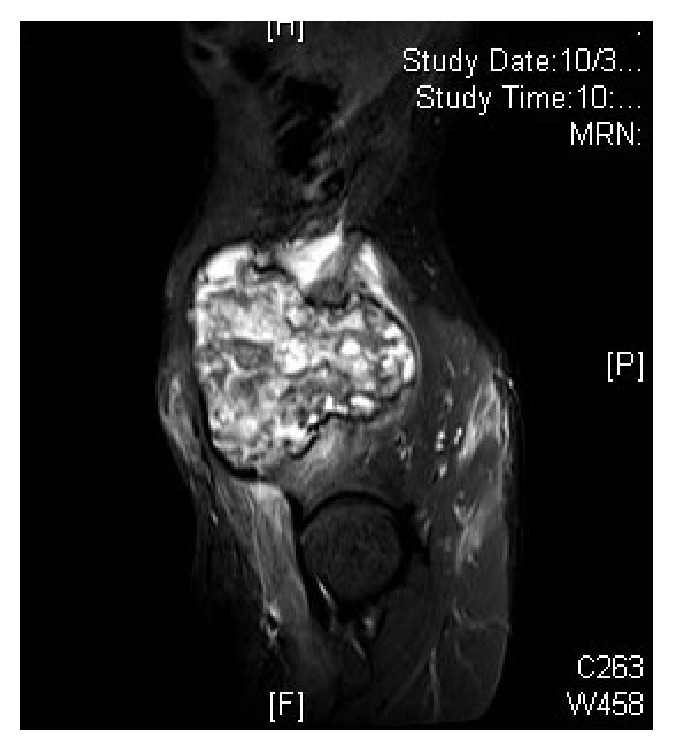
MRI T2W sagittal cuts showing dumbbell shaped mass present on either side of the right ilium.
